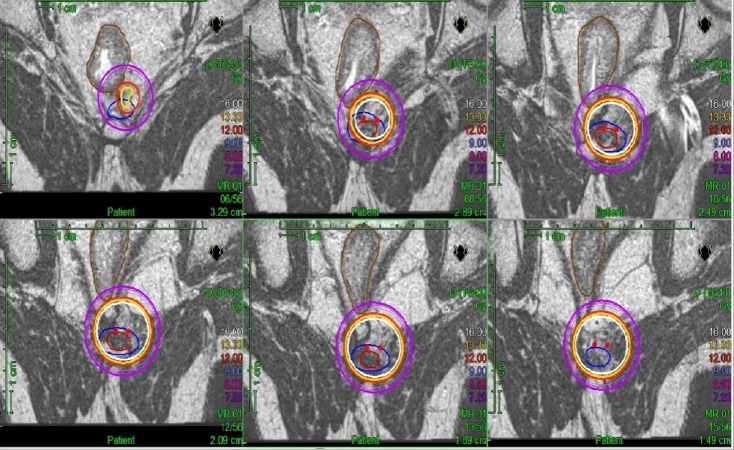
T2-weighted planning MR images (FOV 180 mm, TR 4400 ms, TE 103 ms, 2 mm slice thickness) demonstrating brachytherapy dose distribution in Gy GTV in red, PTV in blue, rectum in brown. MR: magnetic resonance; FOV: field-of-view; TR: repetition time; TE: echo time; GTV: gross tumor volume; PTV: planning tumor volume.
